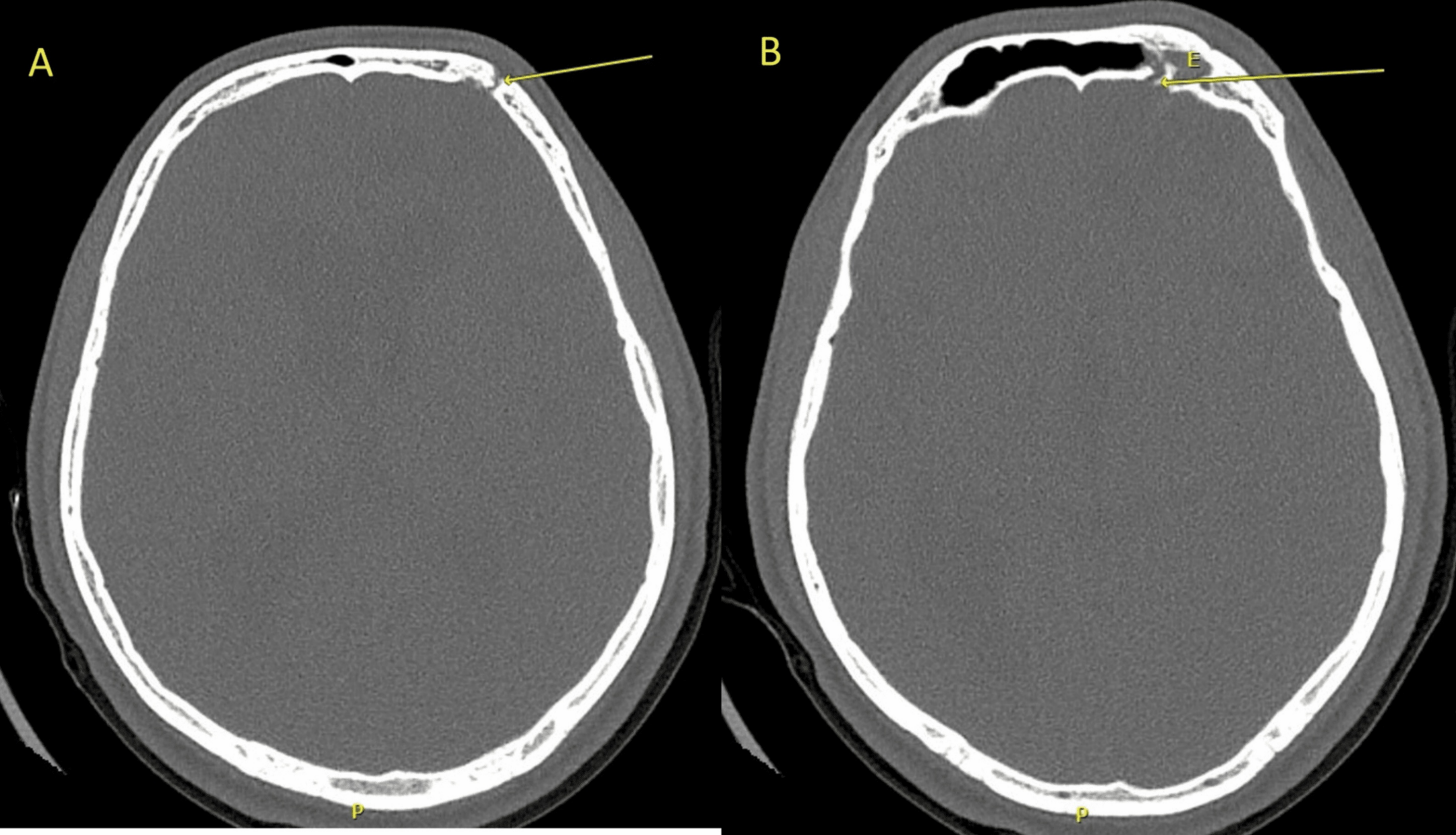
Axial non-contrast cranial CT scan bone window sequence showing the fracture site at the left frontal bone (arrows in sections A and B) with encephalocele (E) protruding the left frontal sinus (in section B).
